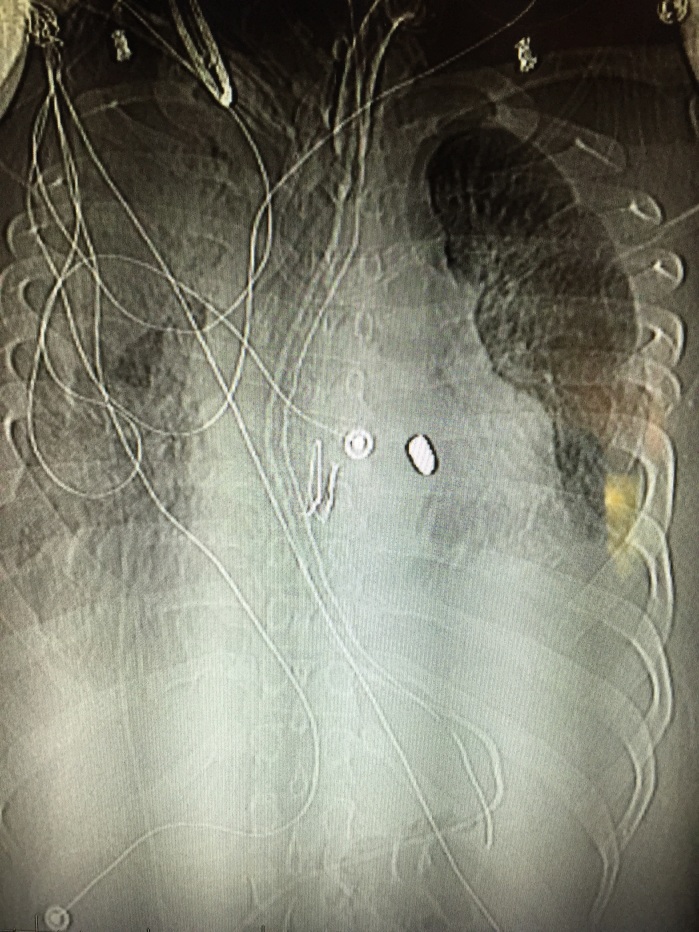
Anteroposteior chest x-ray demonstrating bullet fragment overlying the cardiac shadow.
